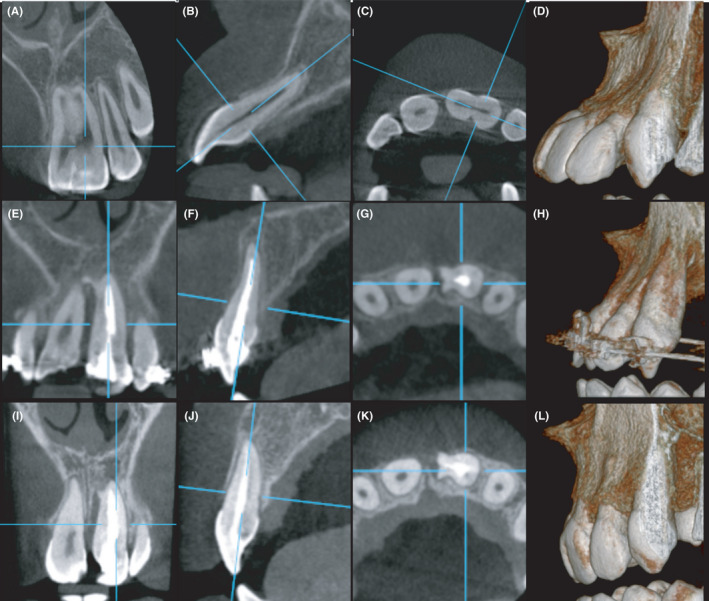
CBCT scans of the patient pre‐operatively in (A‐D), 1 year post‐operative (E‐H) and 4 years post‐operative in (I‐L), where (A, E, I) represent coronal slices; (B,F,J) represent sagittal slices; (C,G,K) represent axial slices; and (D,H,L) represent 3D volume renderings from the profile view
